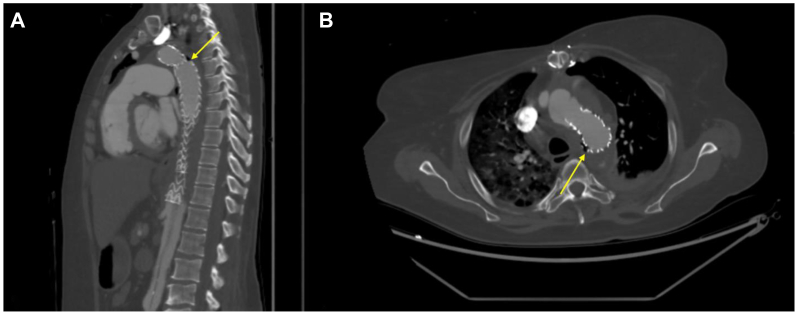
Pre-repair computed tomography (CT) scan of the chest. (A) Sagittal view showing extraluminal gas (*yellow arrow*) at the level of the mid-descending aorta extending toward the esophagus worrisome for aortoesophageal fistula (AEF). (B) Axial view of the adjacent gas (*yellow arrow*).
